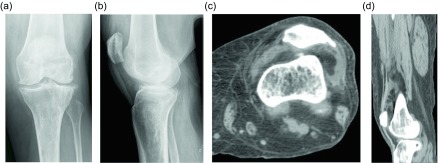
Lipoma arborescens: 59-year-old female with chronic left knee pain. Frontal (a) and lateral (b) radiographs demonstrate tricompartamental osteoarthrosis with fullness of the suprapatellar recess. Axial (c) and sagittal (d) non-contrast CT images reveal a suprapatellar joint effusion with internal small, lobulated masses of fat-attenuation.
